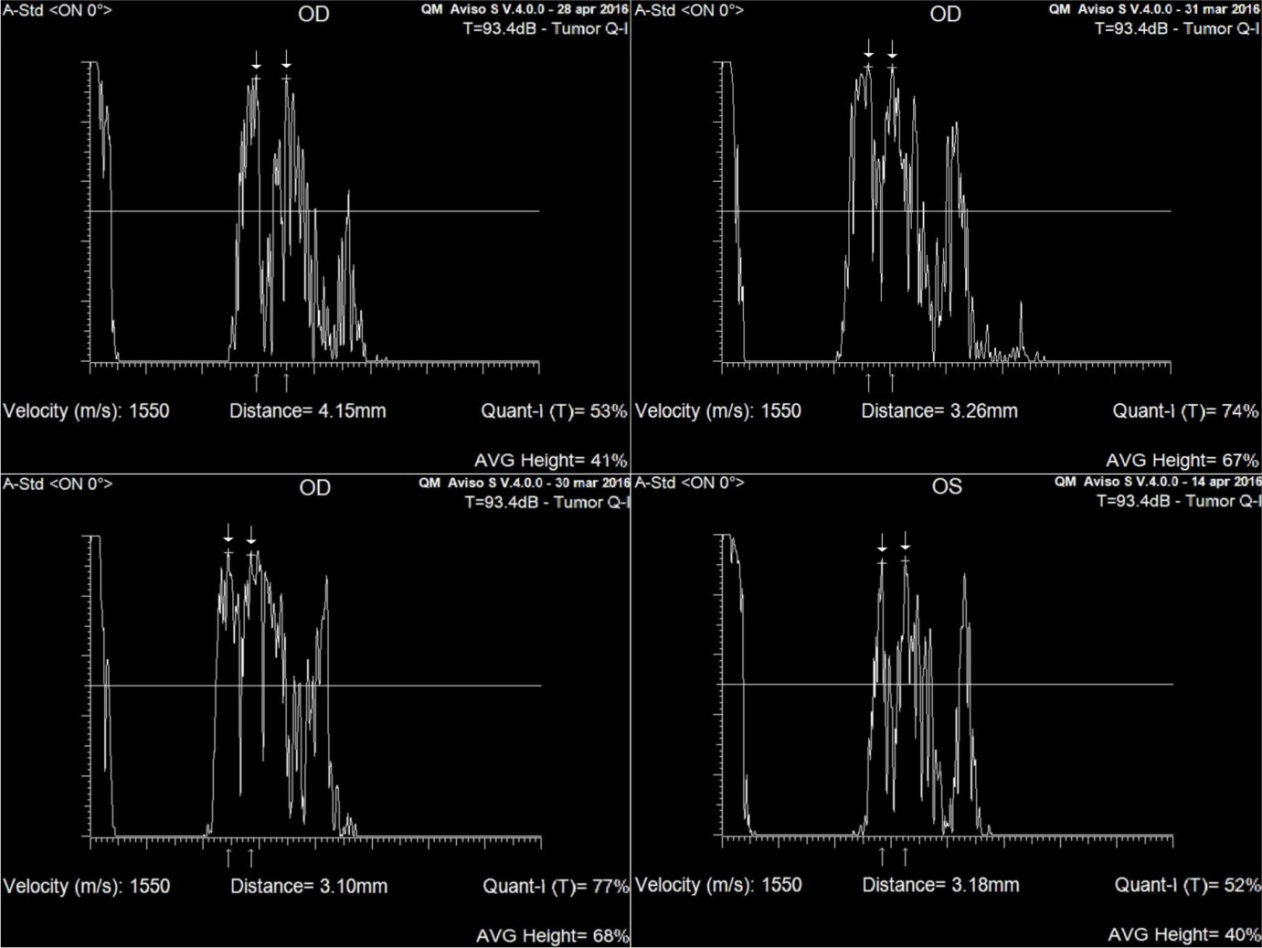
Echograms showing optic nerve subarachnoid space (ONSAS) measurements, using the standardized A-scan ultrasound in control group 1 and 2 in top row (left and right panel, respectively) and in glaucomatous group 1 and 2 in bottom row (left and right panel, respectively). The arrows over the peaks of the two high and perpendicular spikes, as shown in echograms, indicate the maximum arachnoid space diameter measured in the anterior third of the optic nerve.
